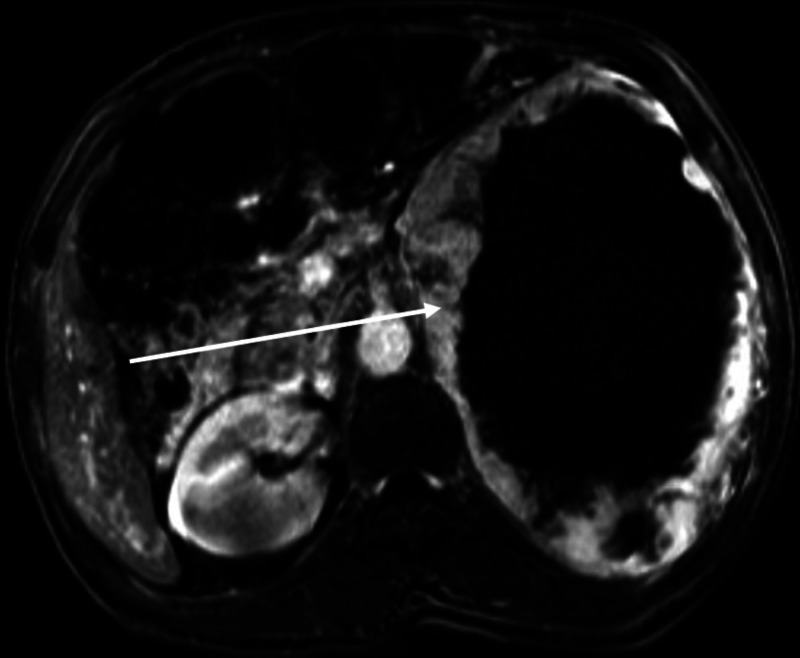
Abdominal magnetic resonance imaging (axial image) showed a large centrally necrotic, heterogeneous, and peripherally enhancing mass (arrow) in the left upper abdomen that measured 15.9 × 12.7 × 14.7 cm.
